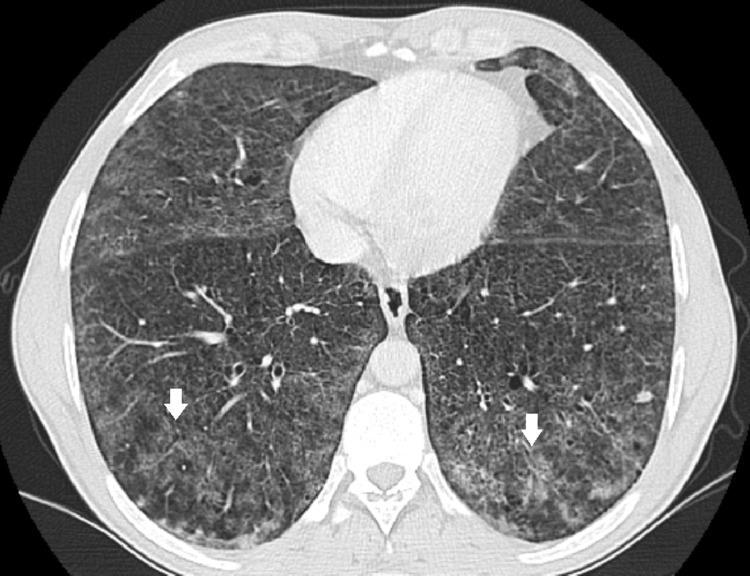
CT scan showing bilateral lower lobes ground glass opacities associated with intermixed interlobular lines in lower lobes (white arrows).
